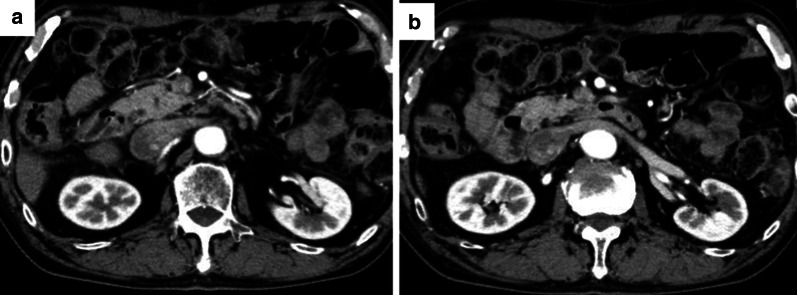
CT findings 7 months after surgery. a, b The para-aortic lymph nodes maintained a reduced state
